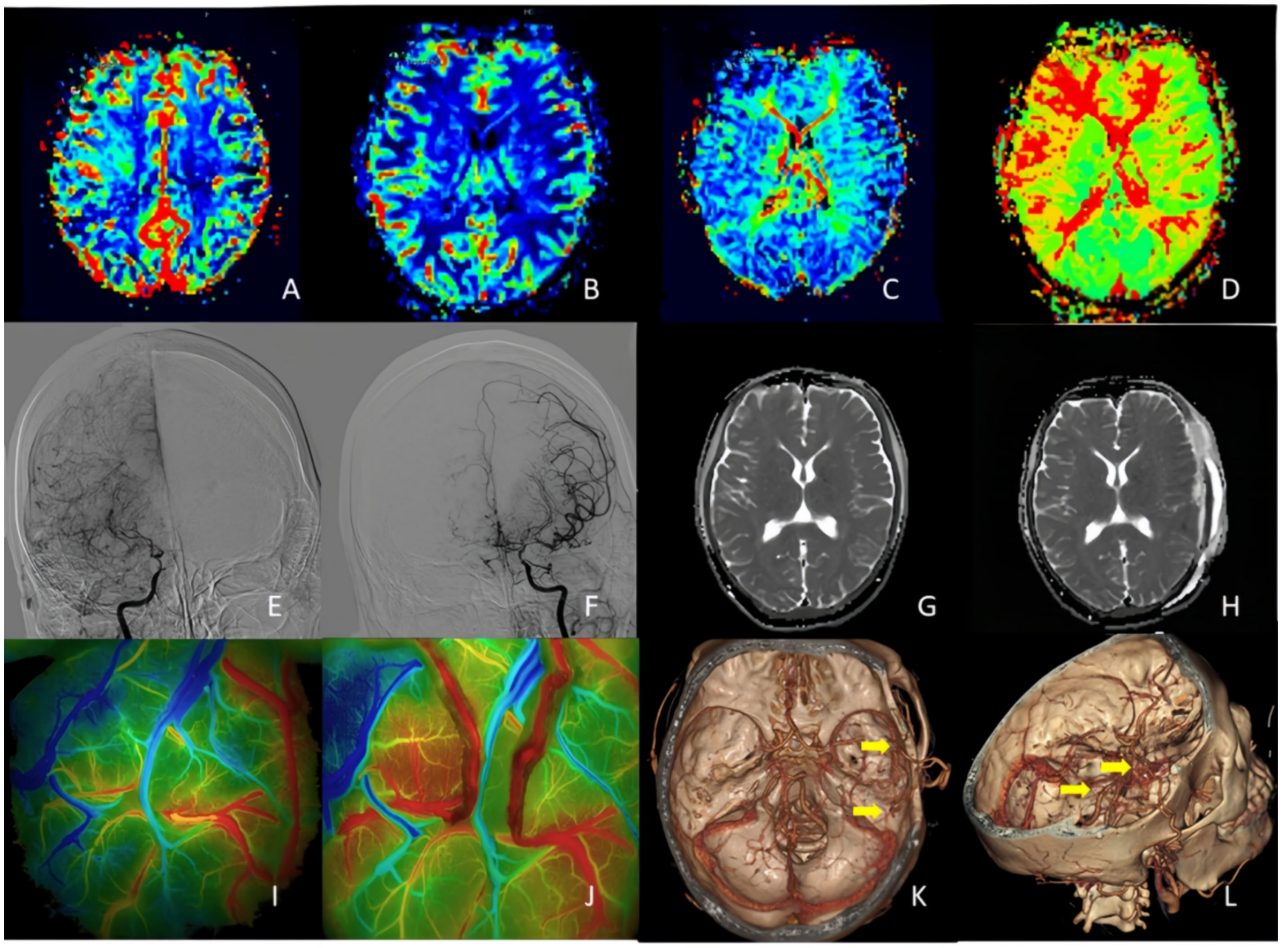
A 32-year-old woman who presented with right side hemiparesis that gradually worsened. Postoperatively, on day 4 the patient developed transient motor aphasia speech impairment which improved at 10 day. (A,B) PWI indicated that bilateral CBV and CBF were symmetrical, and no significant increase or decrease was observed. (C,D) PMI showed MTT and TTP delayed in bilateral occipital lobe and right frontotemporal lobe. (E,F) DSA indicated right anterior cerebral artery and right middle cerebral artery occlusion with moyamoya vessels and severe stenosis of the left middle and anterior cerebral arteries. (G,H) On postoperative day 4, follow-up MRI performed due to transient motor aphasia showed no significant difference as compare to preoperative images. (I,J) FLOW800 angiography results before and after double–branch STA-MCA bypass. (K,L) 3D-CTA reconstruction showed the location of double-branch bypass. ADC, apparent diffusion coefficient; 3D-CTA, three-dimensional CT angiography.
